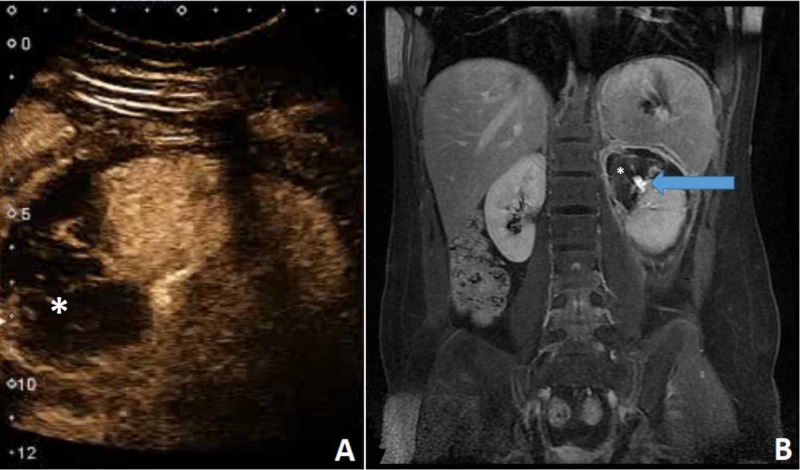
CEUS (A) showed good correlation of vascular defect (asterisk) compared to MRI (B). MRI additionally showed the urinary excretion (arrow). CEUS, contrast-enhanced ultrasound MRI, magnetic resonance imaging
